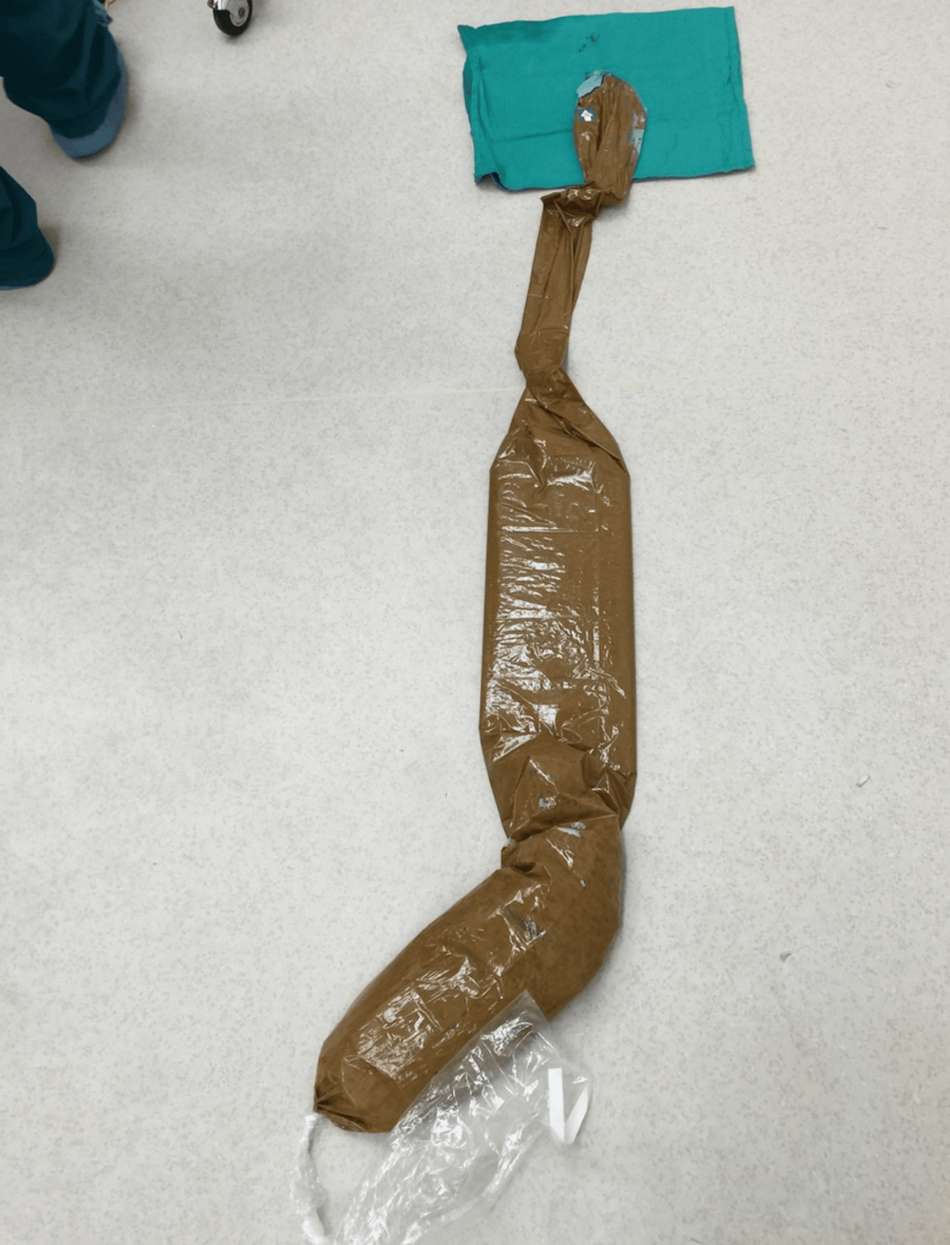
The sterile US sleeve filled with approximately 2 liters of fecal matter after being removed from the operative field US: Ultrasound
